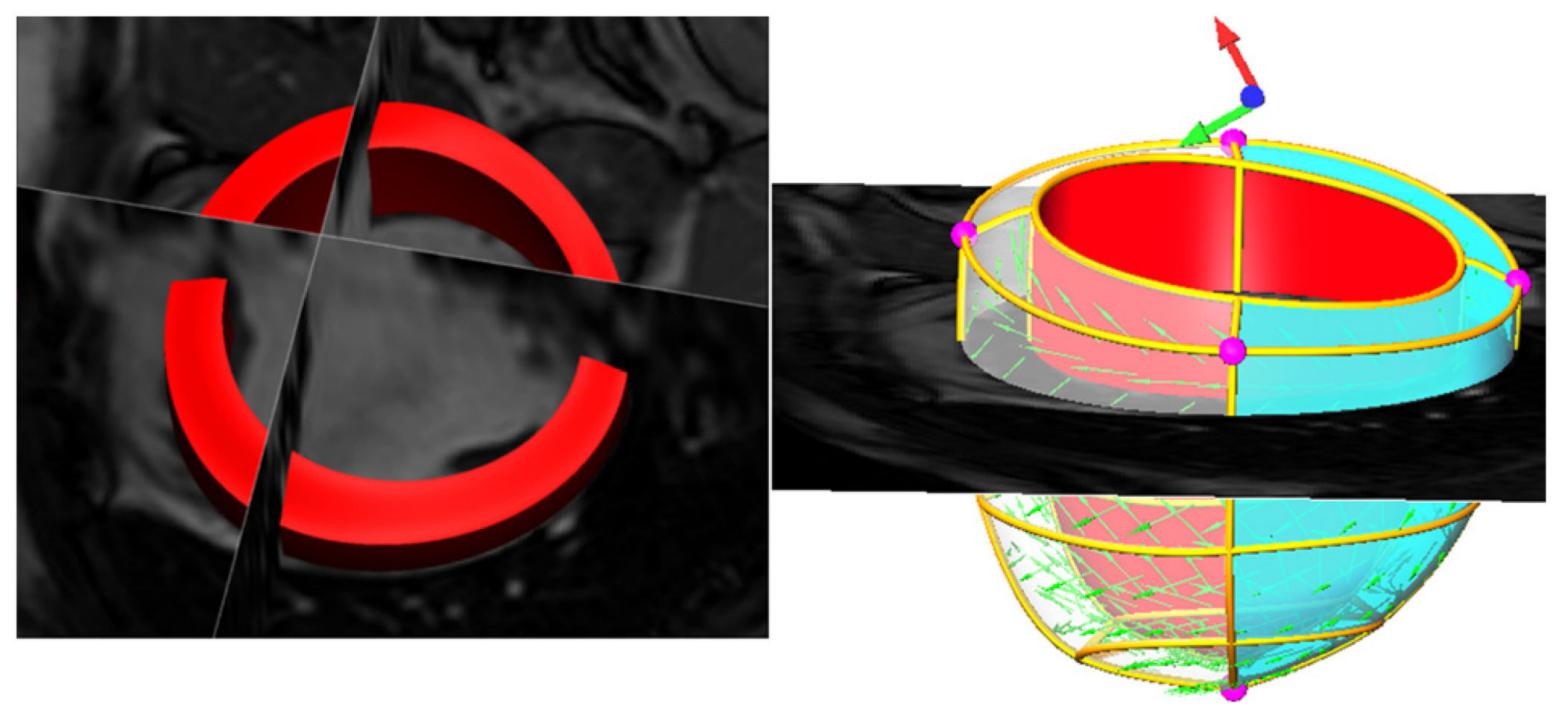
LV volumetric segmentation of the end-diastolic cine MRI (left) and geometric
